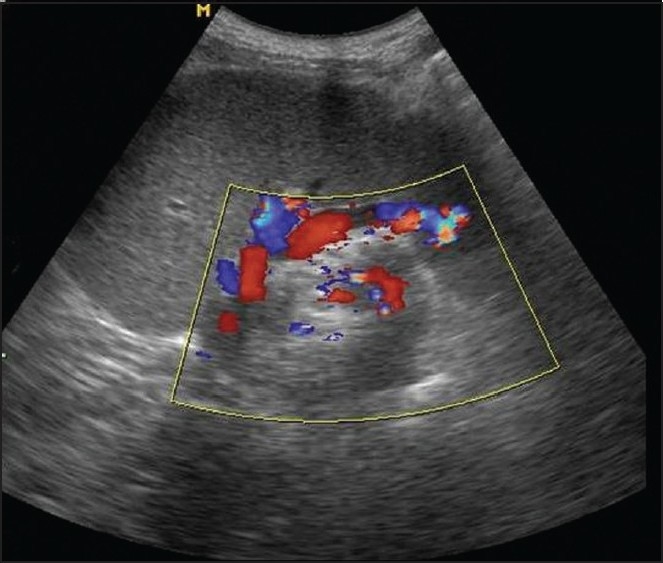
Ultrasonography image demonstrated a whorl of concentric vessels in the region of splenic hilum. Note a spleen of ectopic position
